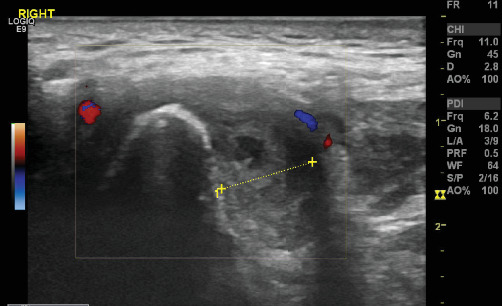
TMJ ultrasound: Intra articular effusion with synovial thickening, the yellow dotted line indicates the periarticular space width; the red and blue doppler signals indicate increased synovial vascularization, which is a sign of inflammation, the bony contour of the condyle looks irregular with signs of erosion.
